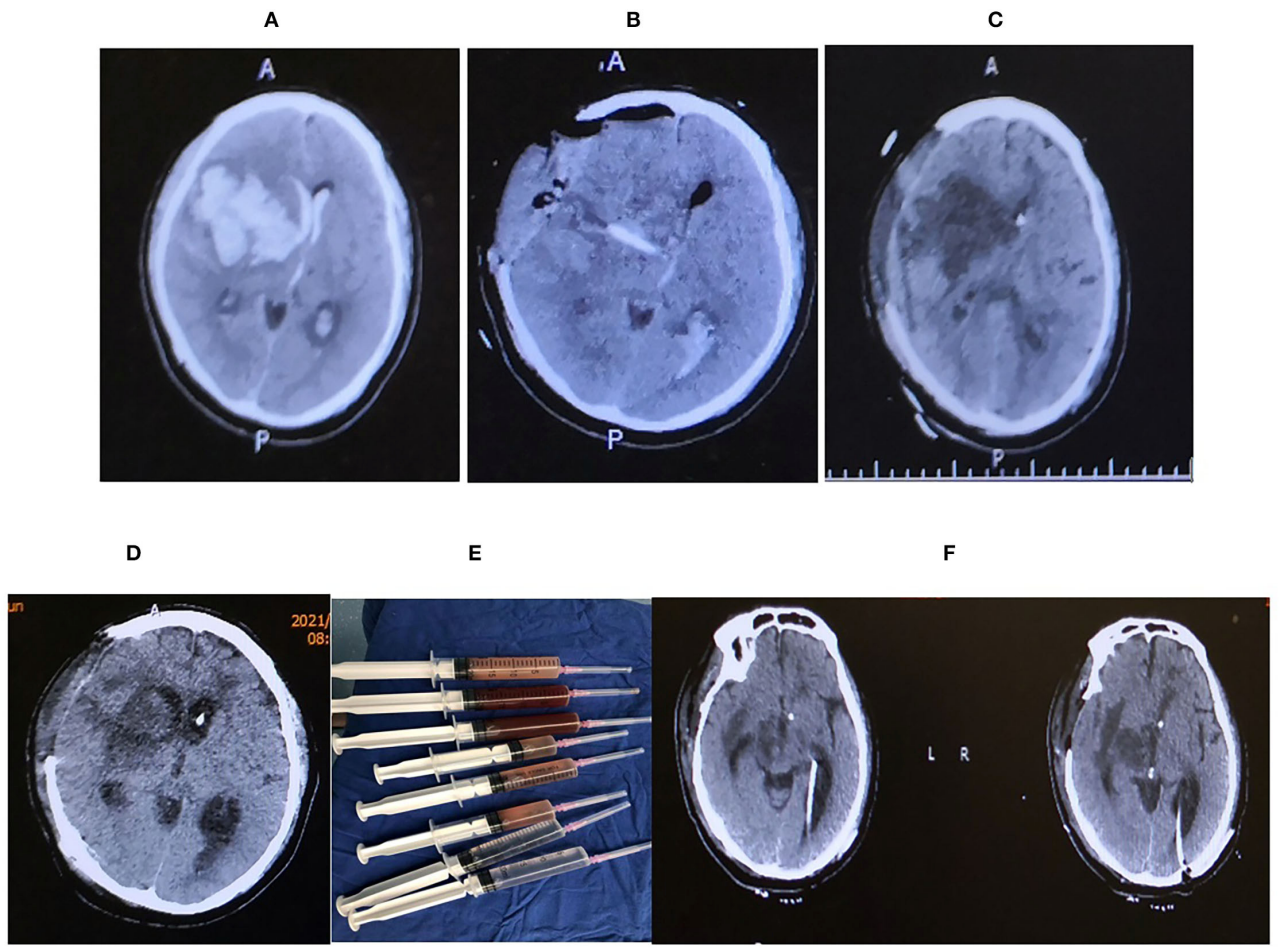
(A) Axial CT showing massive right hemisphere intracranial hemorrhage (ICH) extending to the ventricle with a mid-line shift toward left. (B) The hematoma was evacuated following decompressive craniotomy with intracranial pressure (ICP) probe and external ventricular drainage (EVD) *in situ*. (C) Ommaya pump was placed. (D) Axial CT head showing features suggestive of ventriculitis, (E) irrigation syringe following a thorough ventricular irrigation in the operating room, irrigation was done until the ventricular fluid becomes transparent, (D) Axial head CT after the ventricular irrigation and B/L EVD placement. (F) Axial CT head before discharge.
